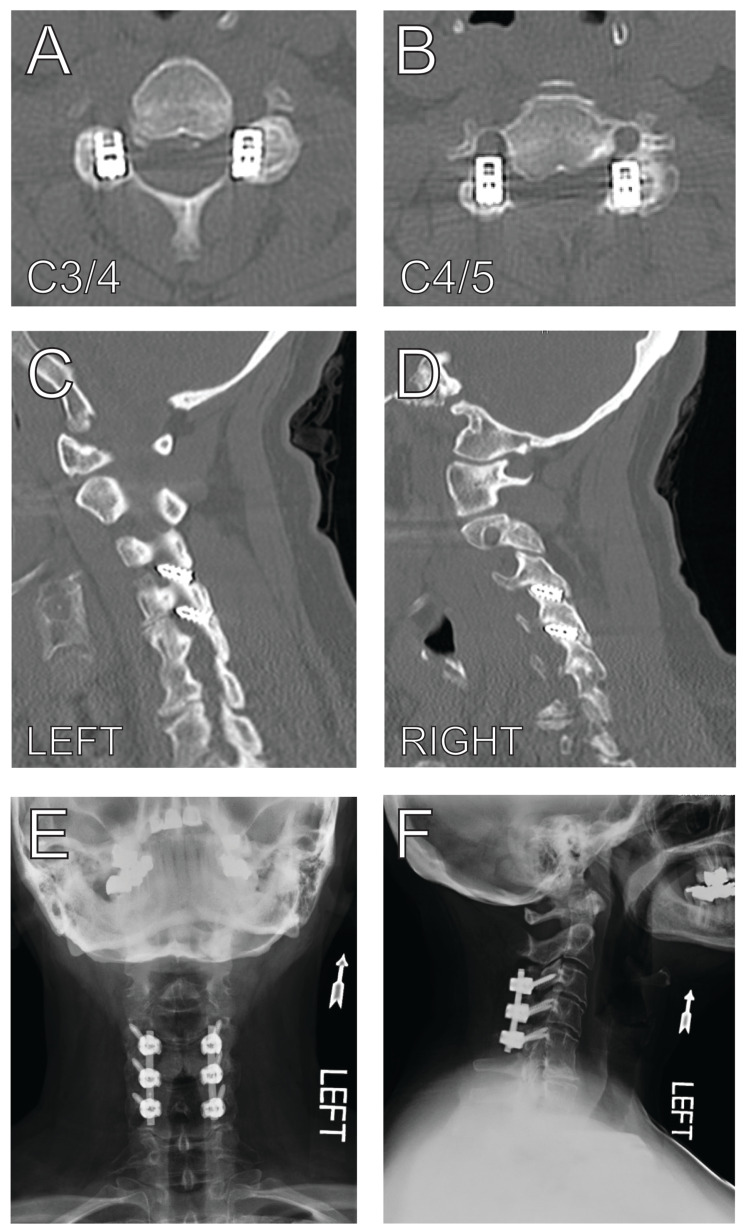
Preoperative and postoperative imaging after removal of malpositioned interfacet implant Axial CT at C3/4 (A) and C4/5 (B) demonstrate implant impingement along the left C4/5 foramen. Sagittal CT along the left (C) and right (D) cervical facets demonstrate impingement of the left C3/4 and C4/5 neural foramen. Postoperative anteroposterior (E) and lateral (F) X-rays demonstrate the successful removal of interfacet implants with the placement of lateral mass fixation from C3-C5 bilaterally.
